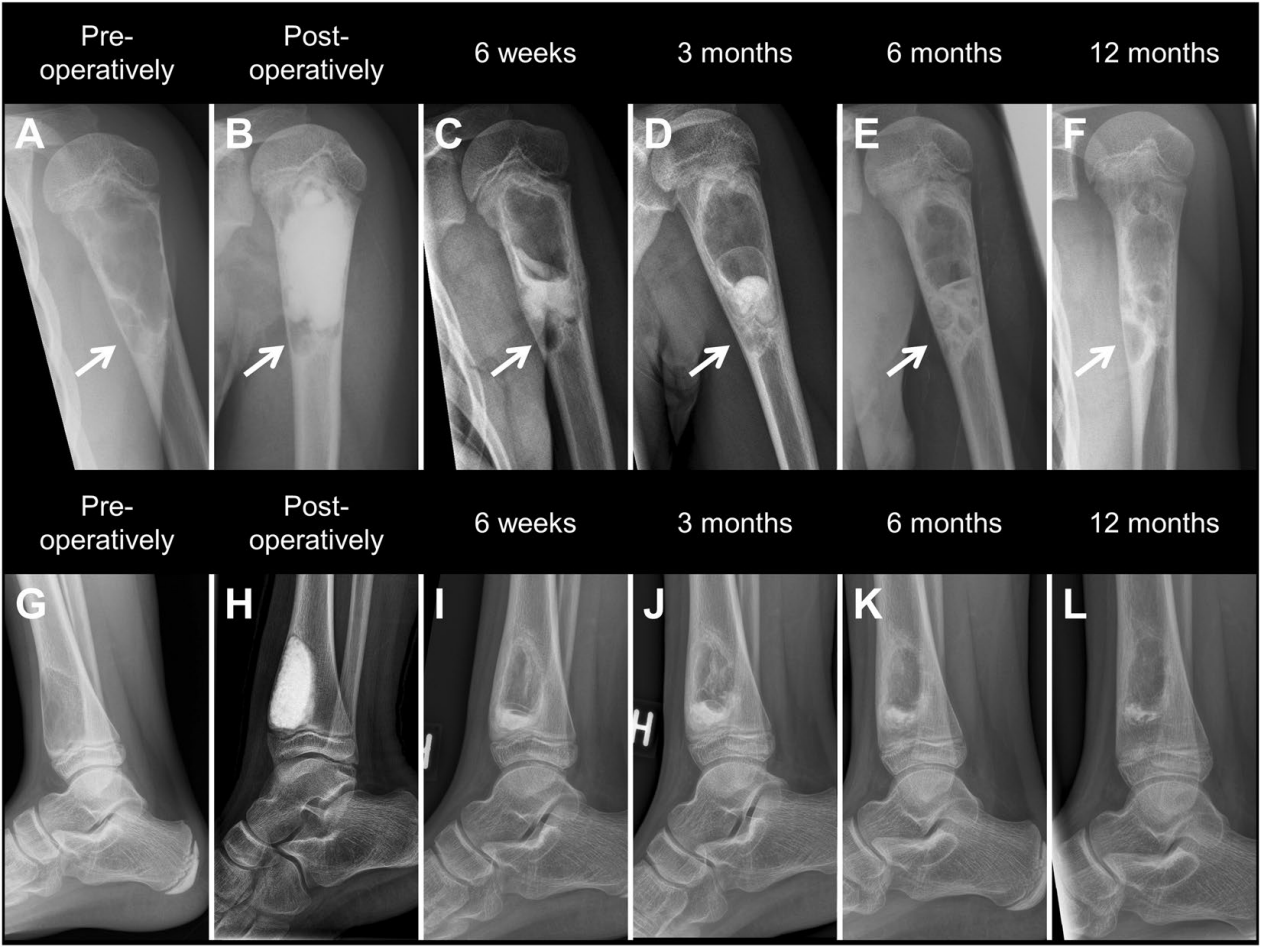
Upper row: Simple cyst of the proximal humerus treated by curettage and defect-filling using CERAMENT|BONE VOID FILLER. (A,B) Pre- and post-operative x-rays showing decreased cortical thickness as well an isolated chamber in the distal part of the affected bone (arrow), which is not accessed or filled during the operation. (C–E) X-rays taken after 6 weeks, 3 and 6 months showing increasing product resorption and cortical thickening. (F) X-ray taken after 12 months showing normal cortical thickness in the main part of the cyst, and reduced cortical thickness in the non-treated distal chamber as a sign of possible local recurrence. Lower row: Aneurysmal bone cyst of the distal tibia treated by curettage and defect-filling using CERAMENT|BONE VOID FILLER. (G,H) Pre- and post-operative x-rays showing decreased anterior cortical thickness and complete defect filling. (I–L) X-rays taken after 6 weeks and 3, 6, and 12 months showing increasing product resorption and cortical thickening. *Note the increasing distance between the growth-plate and the distal aspect of the cyst*.
